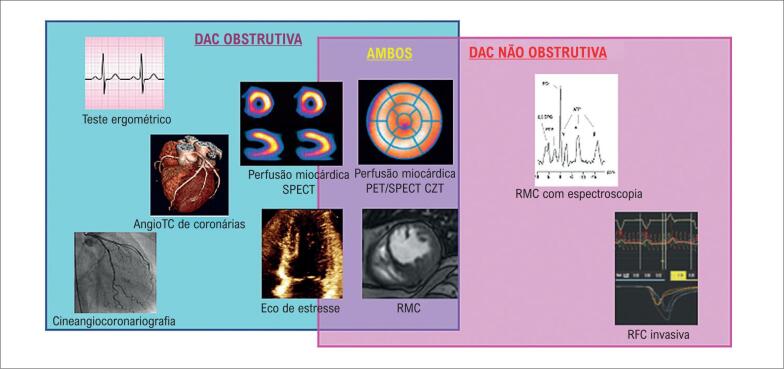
Modalidades para avaliação de doença arterial coronariana obstrutiva e não obstrutiva. AngioTC: angiotomografia; CZT: cádmio, zinco e telúrio; DAC: doença arterial coronariana; PET: tomografia por emissão de pósitron; RFC: reserva de fluxo coronariana; RMC: ressonância magnética cardíaca; SPECT: tomografia computadorizada por emissão de fóton único. Adaptado de Koilpillai et al.^100^
